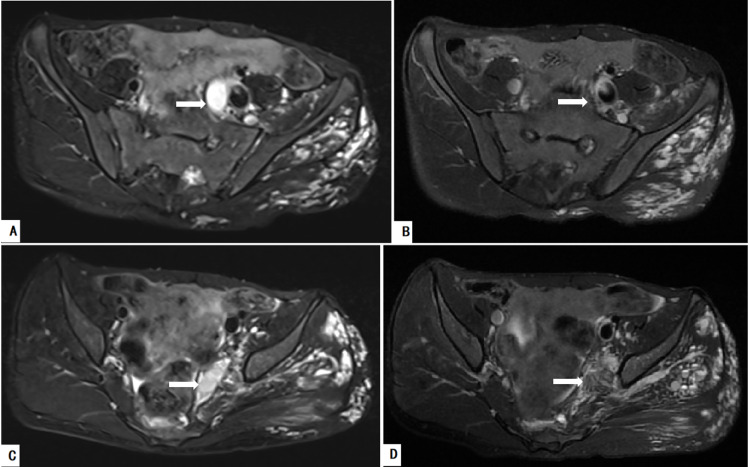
Detecting internal iliac vein on MRI scan preoperatively and during follow-up A and B: A dilated internal iliac vein (arrow) with a high fluid signal on T2-weighted axial MRI scan was successfully ablated (arrow). C and D: Malformed tributaries of the internal iliac vein (arrow) with a high fluid signal on T2-weighted axial MRI scan were also successfully ablated (arrow) MRI: magnetic resonance imaging
